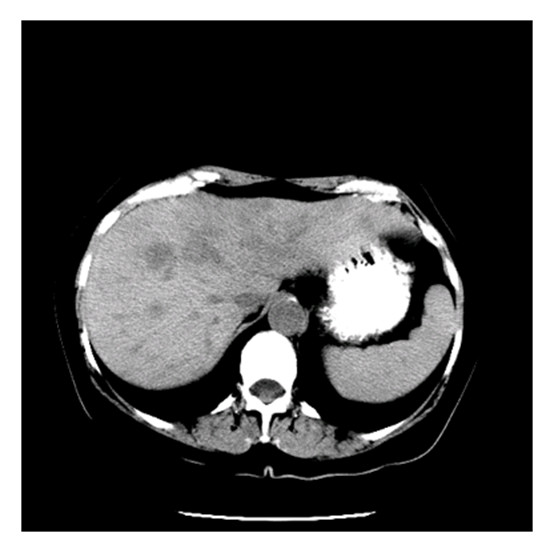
Representative computed tomographic image revealing liver metastases in a PG patient.
